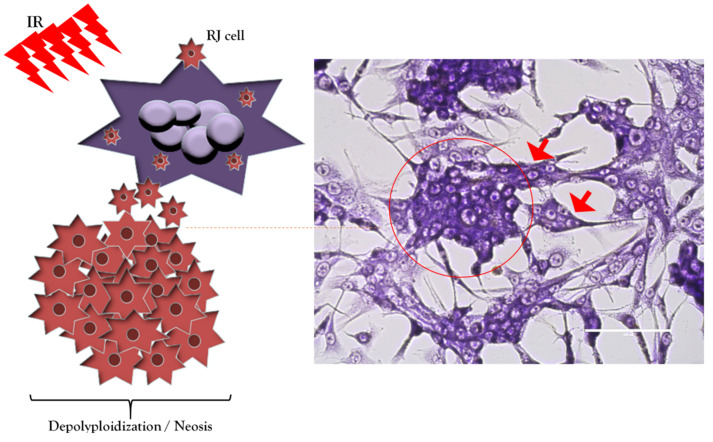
PGCC/MNGC-cell-derived RJ cells in X-ray irradiated GBM U-87 cells.
